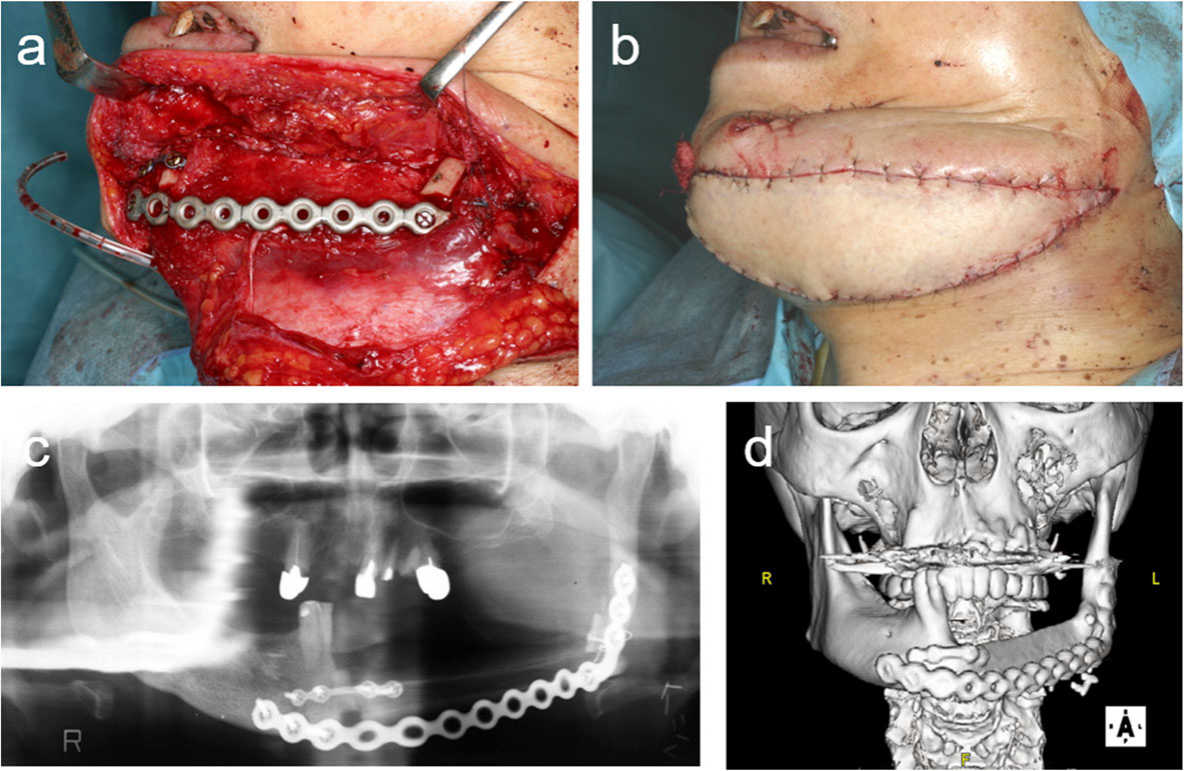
a, b The mandible was reconstructed secondarily with a free vascularized fibula flap 1 year and 7 months after segmental mandibular resection. c Orthopantomogram and d 3-D computed tomography scan after mandibular reconstruction with a free vascularized fibula flap
